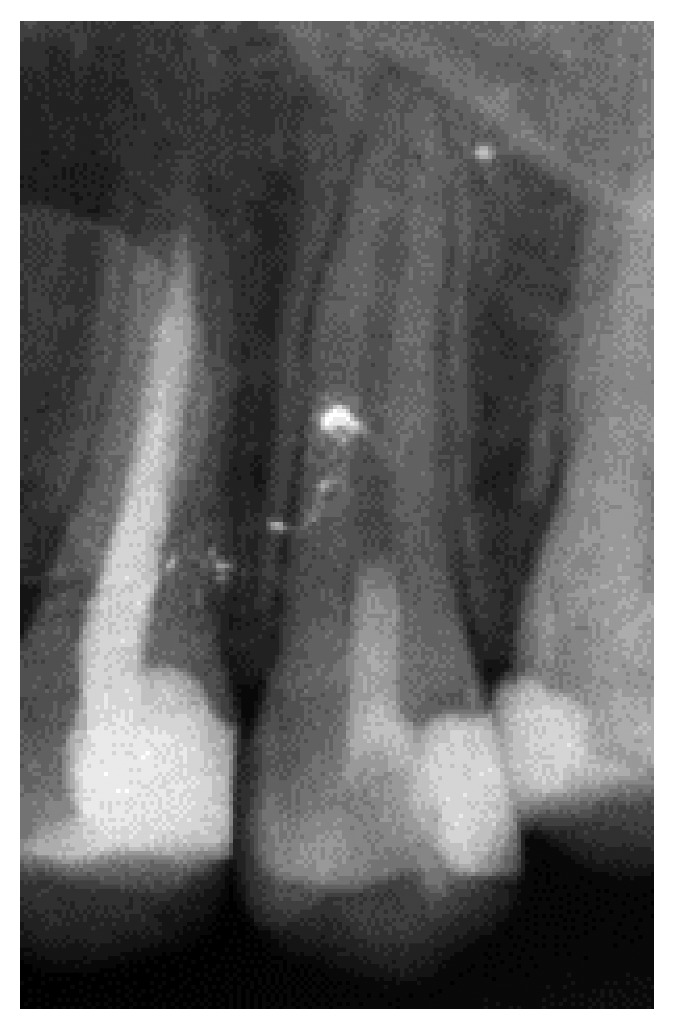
Three-month follow-up radiograph showing a normal periodontal ligament space.
